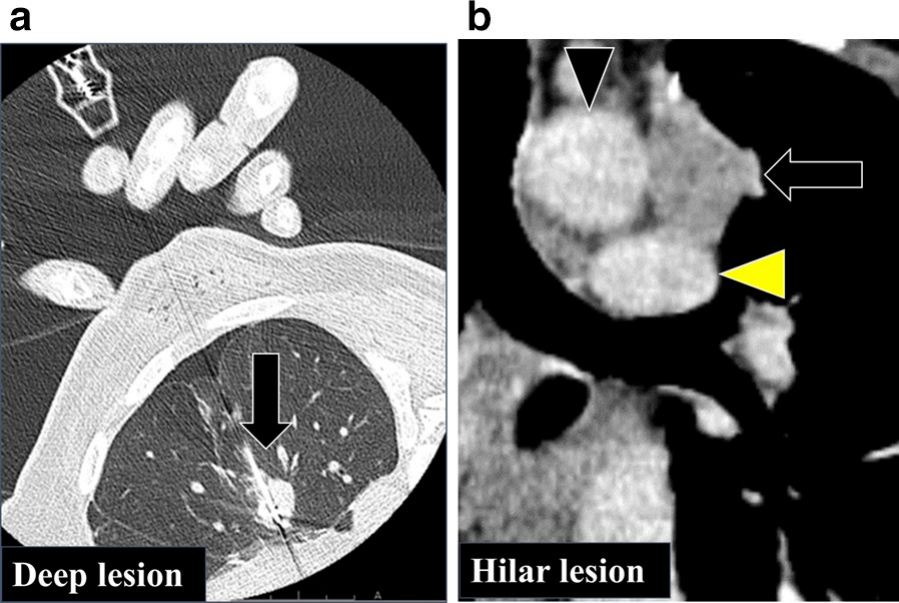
Pretreatment approach for metastatic lesions. Computed tomography revealing a deep pulmonary lesion (arrows), which is treated using radiofrequency ablation (a). Radiation therapy is performed for a lesion near the aortic arch (black arrowhead) and pulmonary artery (yellow arrowhead) (b)
